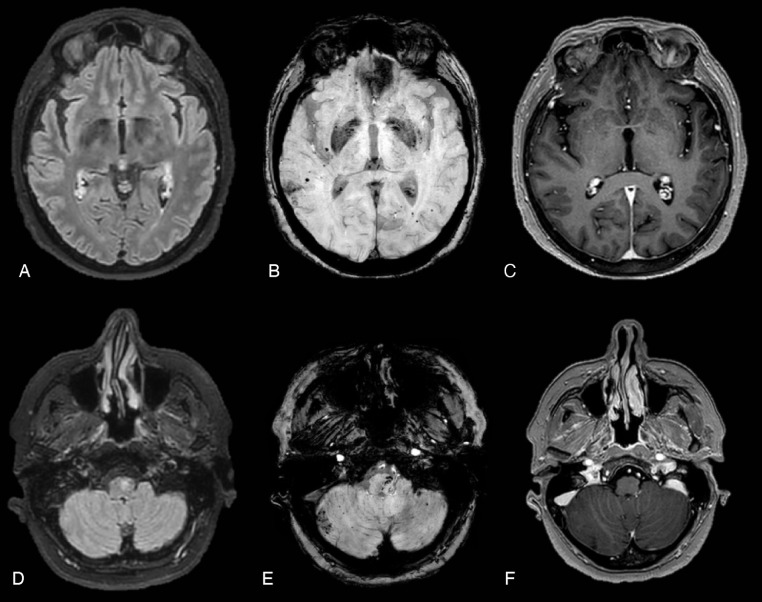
(A, D) FLAIR ax; (B; E) SWI ax; (C-F) post contrast T1 MPRAGE ax. Seven months later a brain MRI disclosed spreading of punctate microbleeds on SWI (B) in cortical and cortical-subcortical areas of both hemispheres, in the brainstem and in the cerebellum. Some of CMBs tended to cluster, leading to macrohaemorrhages, in both temporo-parietal region and in the right inferior cerebellar hemisphere. On FLAIR images (D) WM hyperintensity was observed in the left bulbar pyramid where a serpiginous hypointensity on SWI was detected. No enhancement was seen in supra and subtentorial regions.
